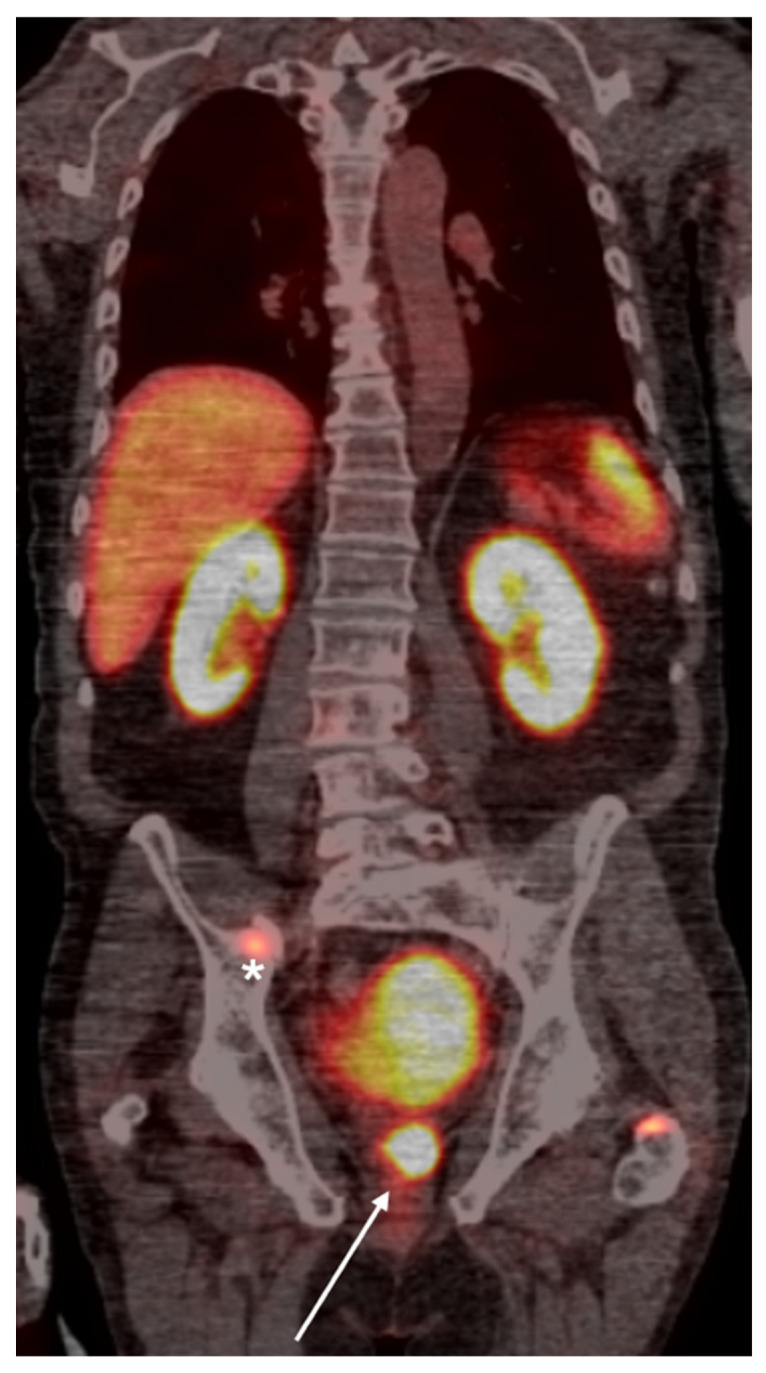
Whole-body PSMA PET scan demonstrating a primary lesion in the left prostate gland (arrow), with evidence of osseous metastasis in the pelvic bones (asterisk).
